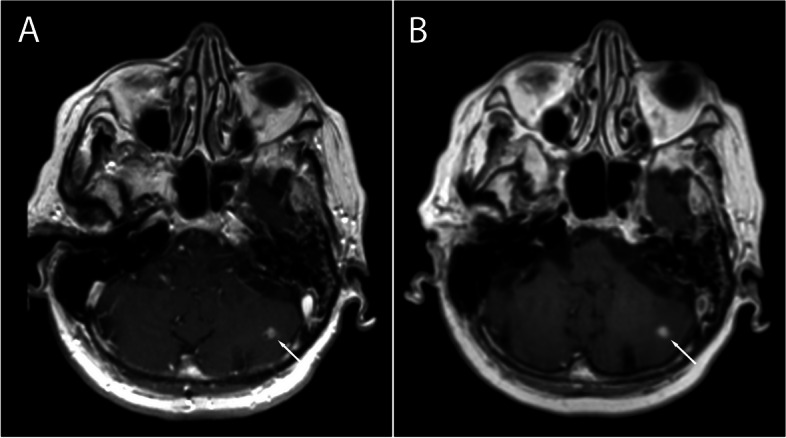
Small metastasis in the left cerebellar hemisphere in an 80-year-old female at 1.5 T, Gadovist 0.1 mL/kg. A T1-weighted SE sequence. B T1-weighted SPACE sequence. The SE sequence was acquired first
